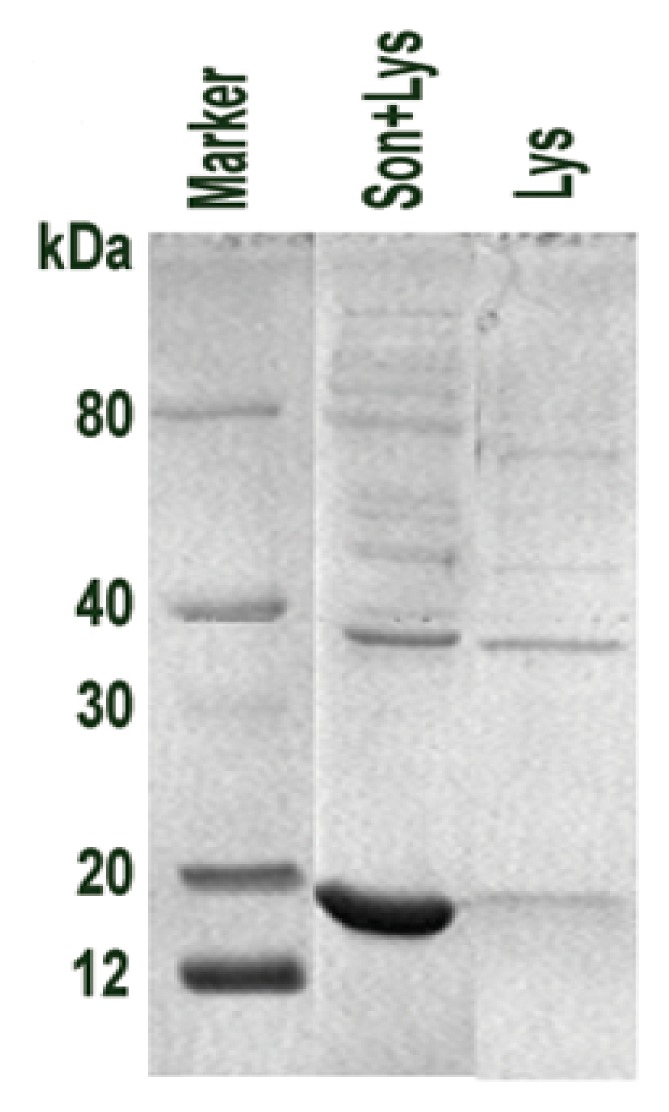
Purification optimization of His-*FIP-fve* fusion protein. The optimized lysis method of recombinant *E. coli* electrophoresed by 12% SDS-PAGE; ultrasonic lysis after lysozyme (Son+Lys) and lysozyme (Lys) methods of *E. coli* with a molecular weight marker. A sample of 3.75 μL of lysis plus 1.25 μL 4× Protein Gel Loading Buffer was used in SDS-PAGE.
